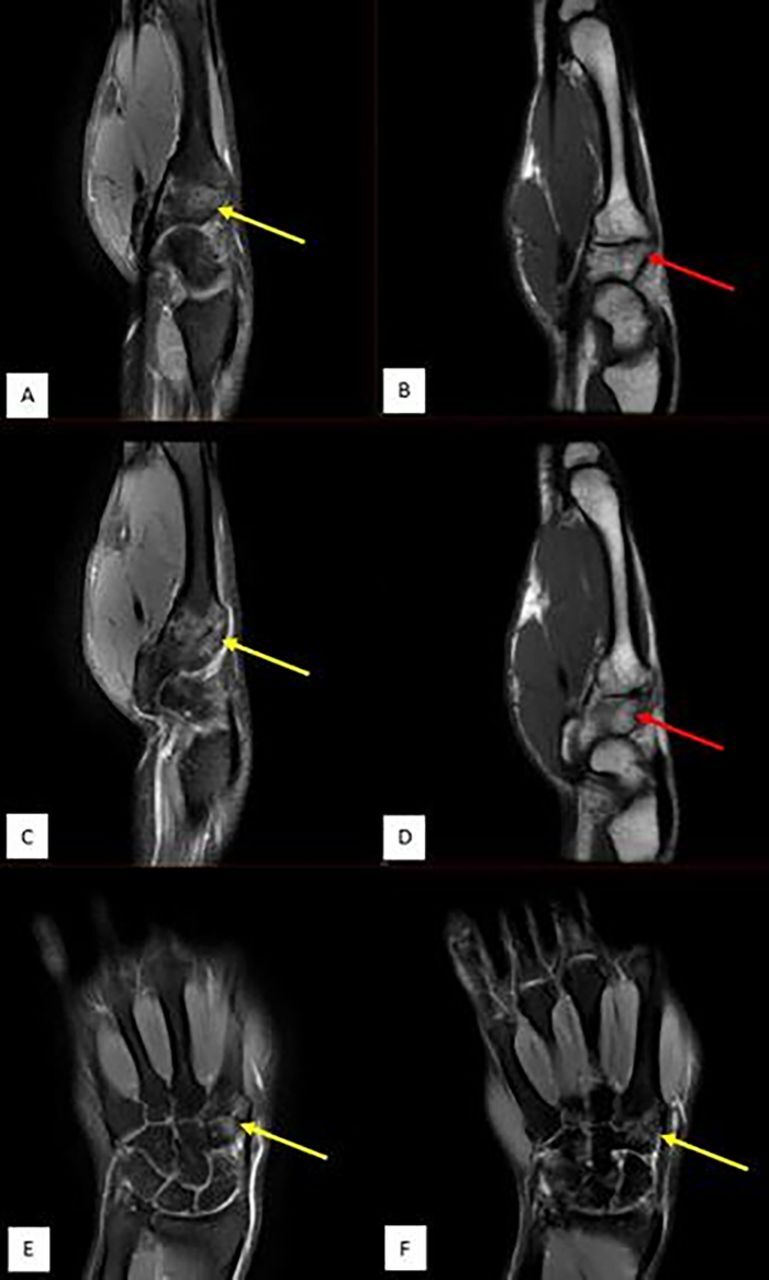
Magnetic resonance imaging of the right wrist—(A and C) sagittal fat suppressed proton density (PD FS), (E and F) coronal PD FS, and (B and D) sagittal T1 images—showing bone marrow edema on opposing sides of the second carpometacarpal joint, predominantly involving the radial aspect of both trapezoid and base of the second metacarpal bones (yellow arrows). Imaging shows no evidence of periosteal reaction or low T1 fracture line (red arrows show only ill-defined edema), and findings are in keeping with a microtrabecular injury.
